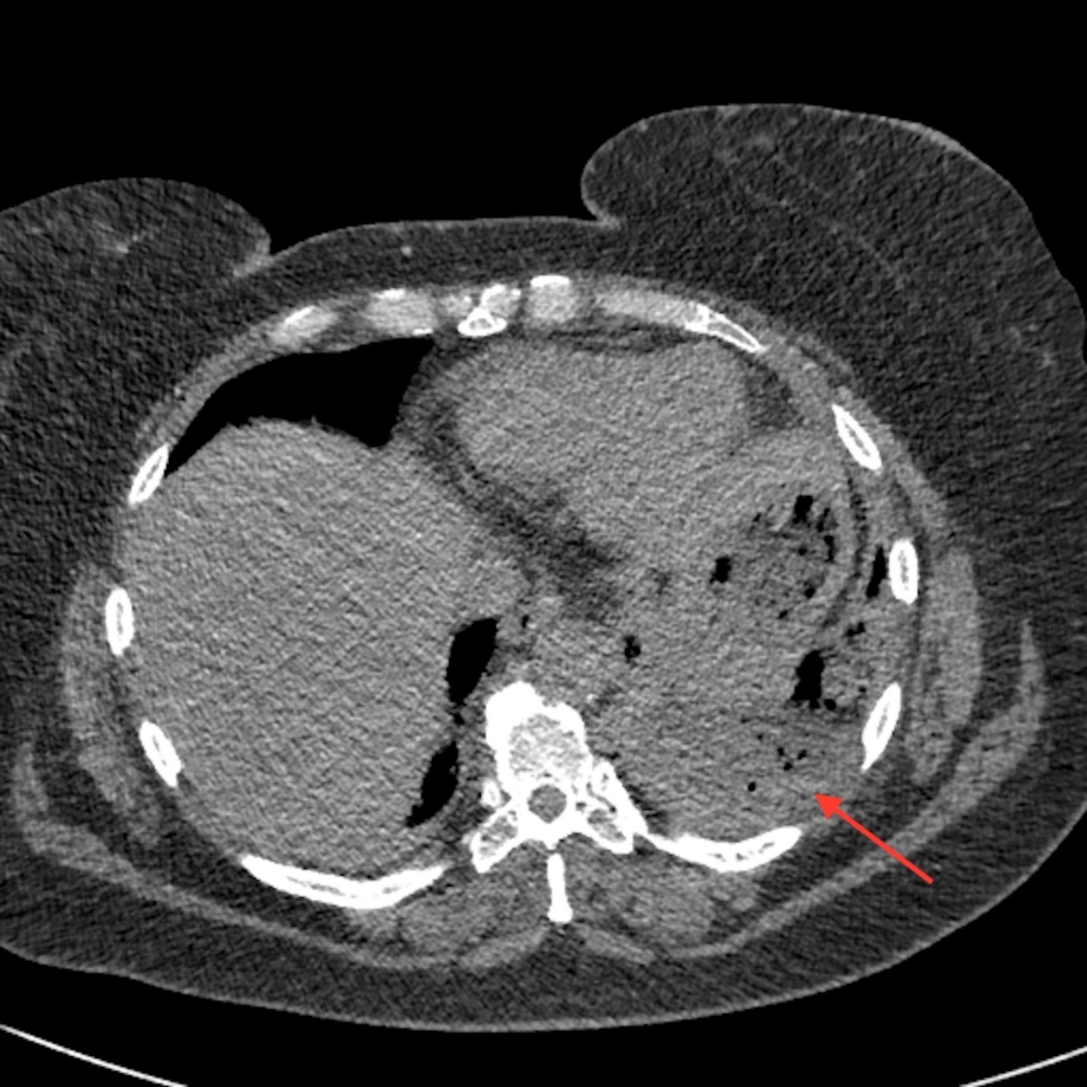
CT chest without contrast performed six days after chest tube placement reveals a collection of mottled foci of gas in the left upper quadrant below the diaphragm (arrow), representing a sub-phrenic abscess and/or a trans-diaphragmatic pleural fistula CT: computed tomography
